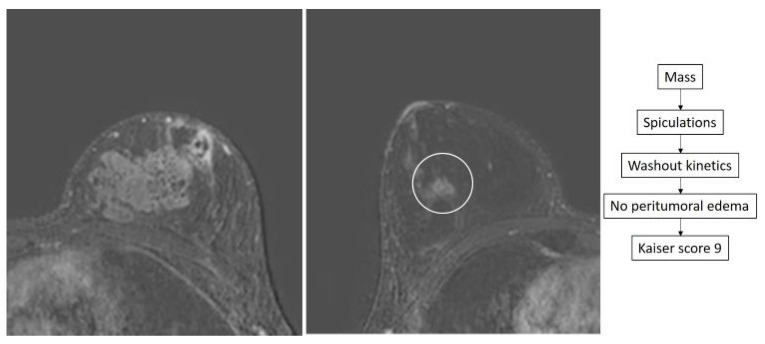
False positive case of a 25-year-old woman with recent diagnosis of locally advanced left breast cancer. Staging breast MRI shows an irregular mass (circle) in the contralateral breast showing some spiculations and exhibiting washout kinetics. The Kaiser score of this lesion was 9. A biopsy was performed showing intraductal papilloma and sclerosing adenosis.
